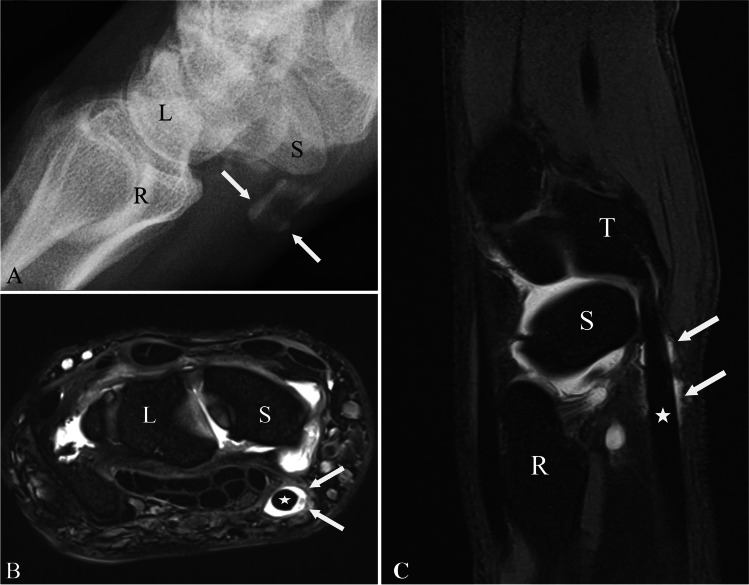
Contrast media in the tendon sheath of the flexor carpi radialis tendon. Fluoroscopic image (a) of contrast media in the tendon sheath of the flexor carpi radialis tendon (white arrows) after midcarpal contrast media injection as an indirect sign of a tear of the STT ligament complex. Corresponding axial (b) (proton density with fat saturation) and sagittal (c) (T1 weighted with fat saturation) MR images also show contrast media (white arrows) in the tendon sheath of the flexor carpi radialis (white star). Abbreviations: S, scaphoid; L, lunate; R, radius; T, trapezium
